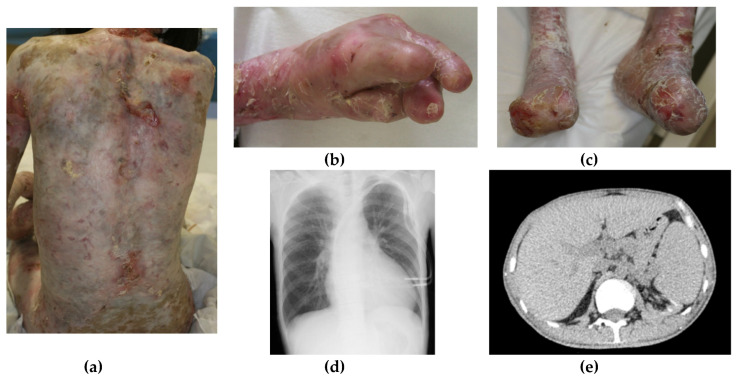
A 37-year-old severe recessive dystrophic epidermolysis bullosa patient is presented. (a–c) Blister and erosion had been observed beginning soon after his birth, resulting in scarring, contraction, and dactylosymphysis of the limbs; (d) a chest radiography revealed cardiomegaly; (e) computed tomography showed hepatomegaly and splenomegaly. The diameter of the aorta is narrow in this patient.
